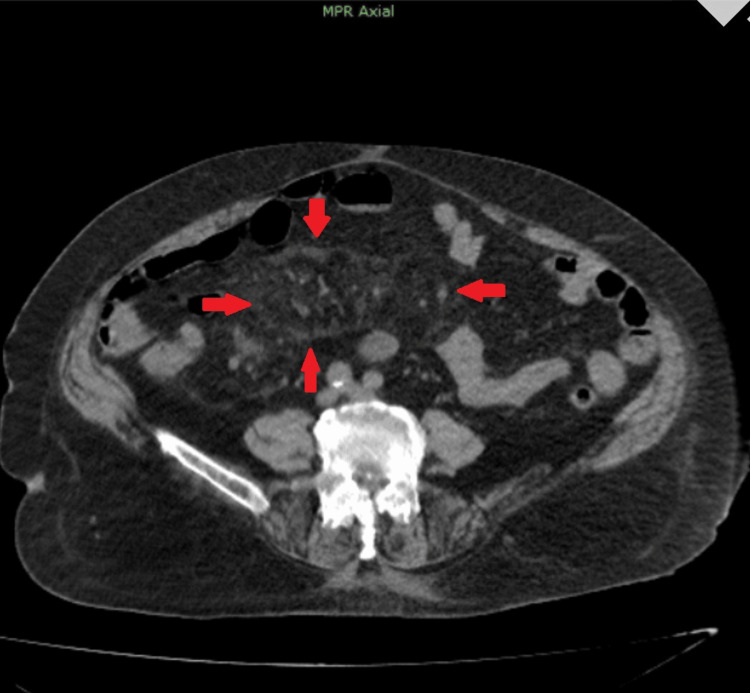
Axial contrast-enhanced CT image showing mesenteric panniculitis Axial contrast-enhanced CT image of the abdomen showing increased attenuation of the mesenteric fat, producing a “misty mesentery” appearance, with associated small subcentimeter mesenteric lymph nodes. These findings are consistent with mesenteric panniculitis. Red arrows indicate mesenteric inflammatory changes and small mesenteric lymph nodes. CT: computed tomography
